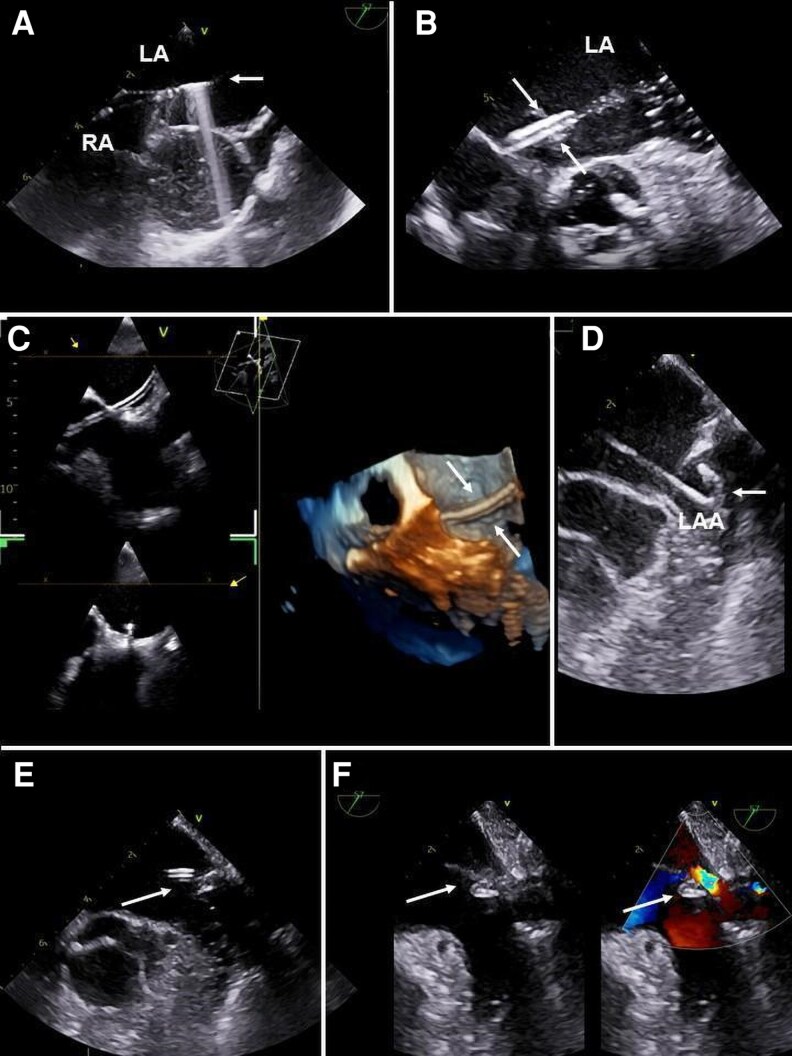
After transseptal access, wires and catheters are advanced into the LA under continuous TOE monitoring, avoiding contact with the atrial wall, aortic wall, or LAA lumen. Once the guidewire reaches the target stenosed PV, the delivery sheath is positioned at its ostium. (*A*) 2D TOE ME bicaval view showing passage of the wire (white arrow) from the RA to the LA. (*B*) 2D TOE superior ME short-axis view demonstrating the catheter (double white arrows) within the LA. (*C*) 3D reconstruction confirming catheter position (double white arrows) in the LA. (*D*) LAA view showing incorrect catheter allocation into the LAA (white arrow). (*E*) LAA view showing correct catheter orientation (white arrow) towards LUPV. (*F*): Simultaneous 2D and colour Doppler view of the LUPV confirming catheter placement (white arrow) within the stenotic LUPV. LA: left atrium; LAA: left atrial appendage; LUPV: left upper pulmonary vein; ME: mid-oesophageal; PV: pulmonary vein; RA: right atrium; TOE: transoesophageal echocardiography.
